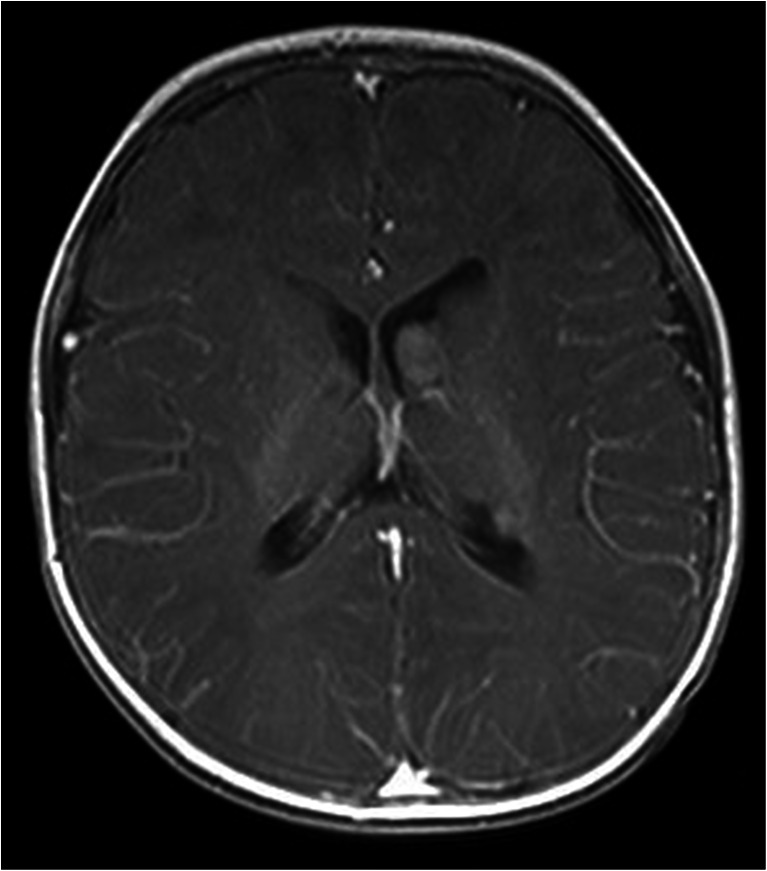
Brain MRI showing SEGA located near the right foramen of Monro and causing hydrocephalus in a 1-week-old patient with TSC
